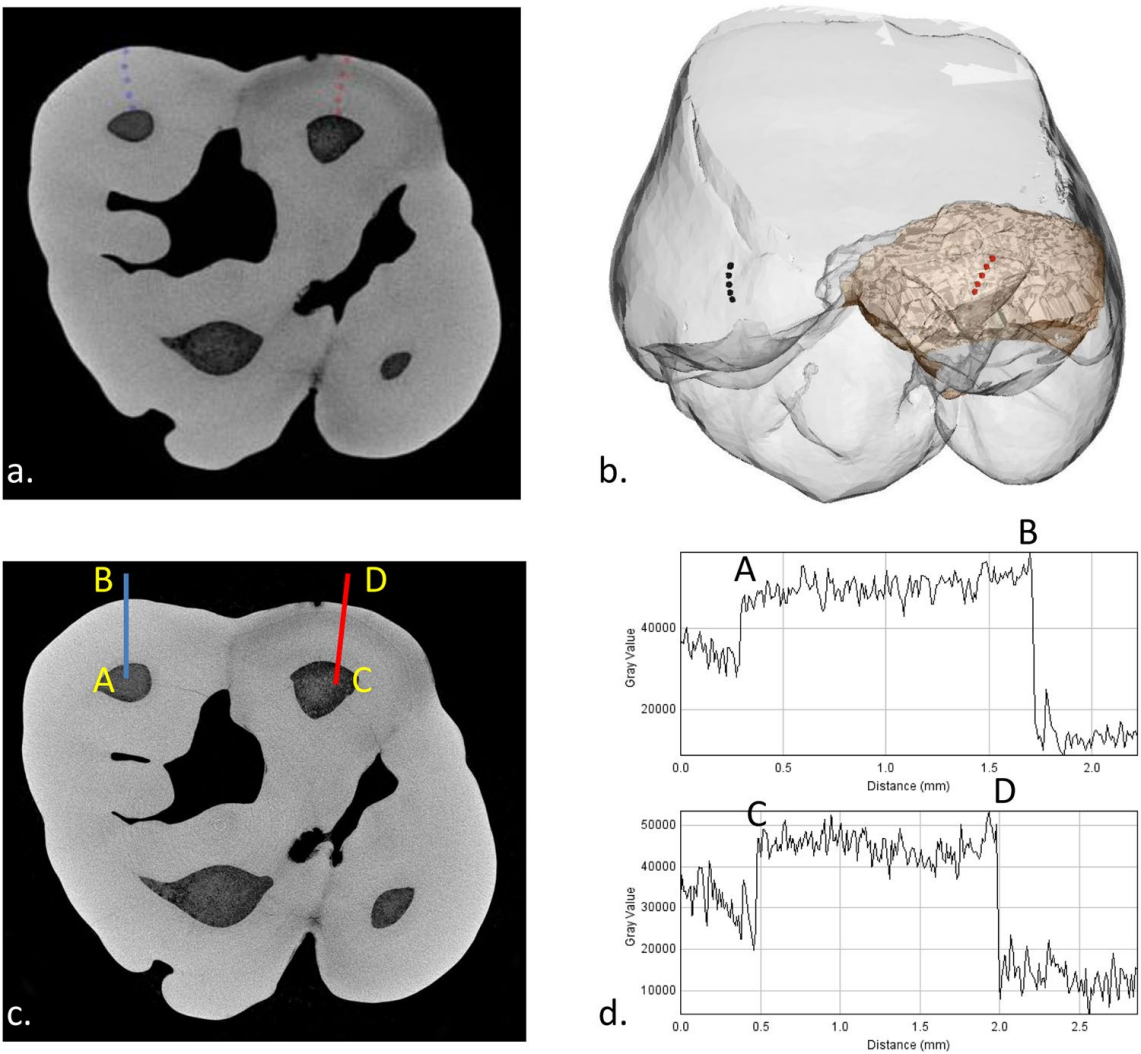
Images of microcomputed tomography analyses in 3D and 2D: (a,b) location of 5 analysis cubes from DEJ to surface on normal (blue cubes) a﻿nd discoloured (red cubes) areas. (c,d) location of profile lines from EDJ to surface through normal (AB) and discoloured (CD) areas.
